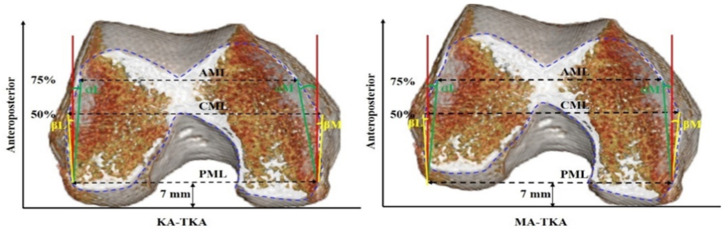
Transverse CT slice at the level of the distal cutting of the femur, indicating the femoral cortical contours (blue). The posterior medial-lateral (PML) width was the posterior femoral condylar cutting width, the central medial-lateral (CML) width was measured at 50% AP, and the anterior medial-lateral (AML) width was measured at 75% AP. Lateral narrowing: αL and βL; medial narrowing: αM and βM.
